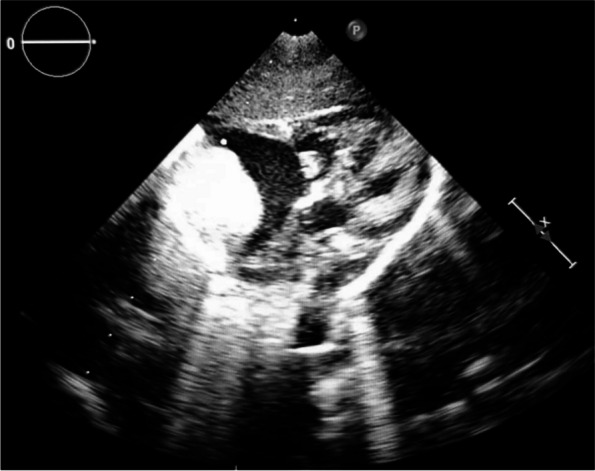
Echocardiogram revealing a hyperechoic, uniform, round 25 mm mass arising from the superolateral wall of the right atrium and protuding into the right atrial cavity, towards the tricuspid valve
